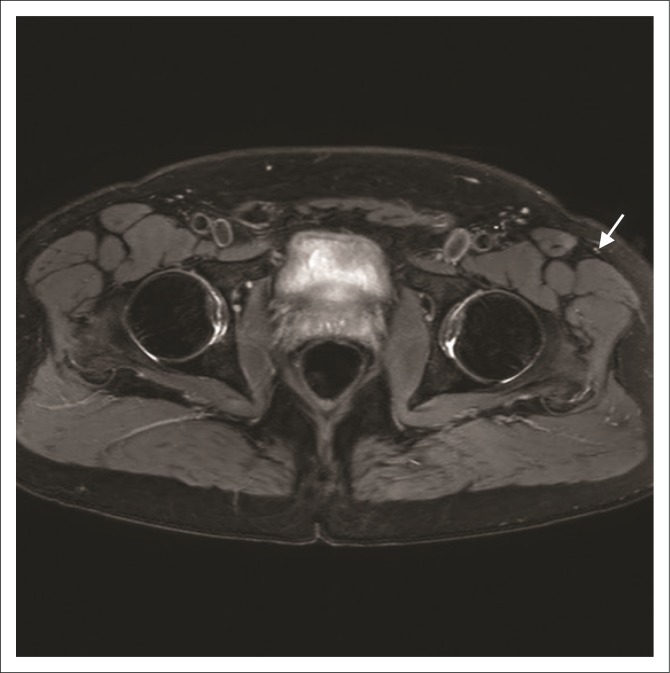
3T proton density fat-saturated axial MR image in the same patient described in Figure 2 demonstrates the persistent high signal of the lateral femoral cutaneous nerve in the subcutaneous tissue where it divides into anterior and lateral branches.
